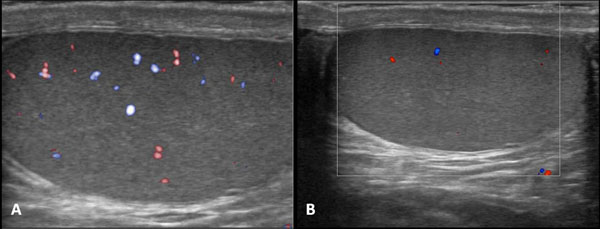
(a,b) Gray-scale and Colour Doppler of testicular torsion. In the early phases of torsion (1–3 hours), testicular echogenicity appears normal. With progression, enlargement of the affected testis and increased or heterogeneous echogenicity are common findings. A definitive diagnosis of complete testicular torsion is made when blood flow is visualized on the normal side but is absent on the affected side .Incomplete torsion refers to cord twisting of less than 360°, in which some arterial flow persists in the affected testis .
